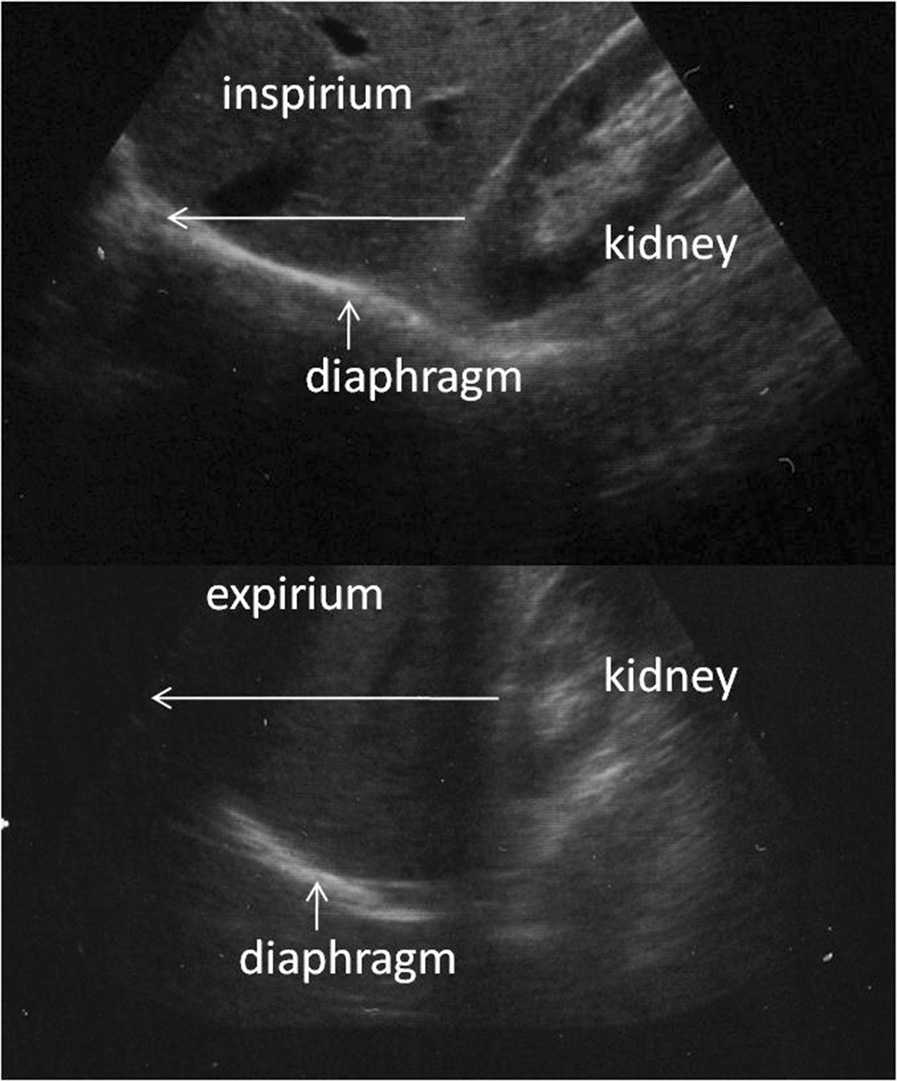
Ultra-sonographic measurement of diaphragm movements. Craniocaudal ultrasound image of the right diaphragm during inspiration (top) and expiration (bottom). Hemidiaphragm movements are measured as shown (arrows).
